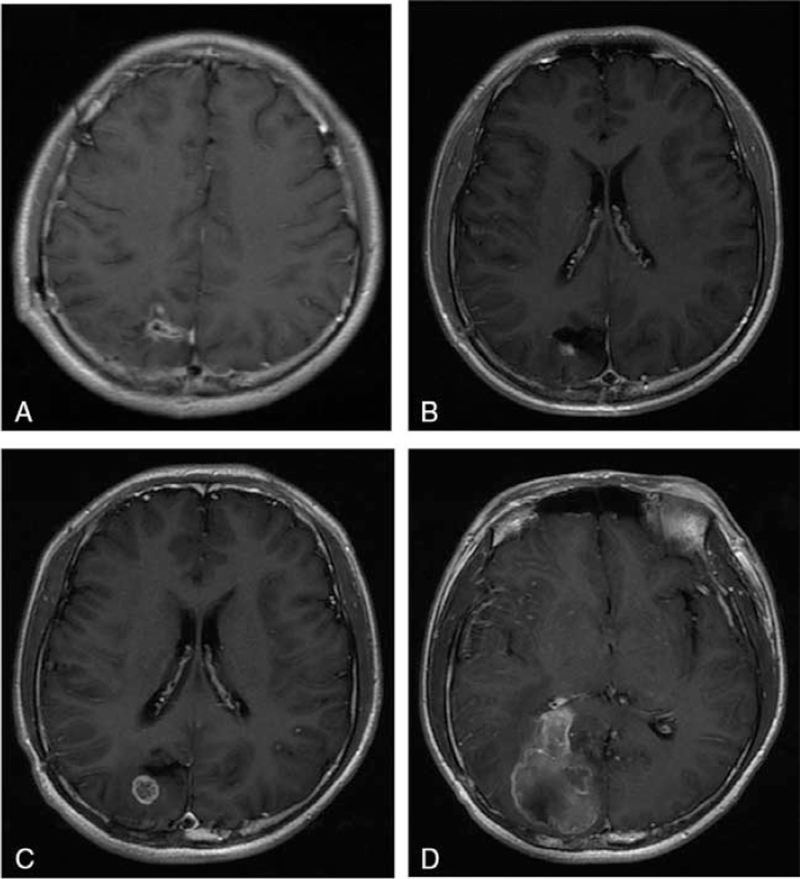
Postoperative imaging. A, Brain MRI obtained at first time after operative demonstrating gross total resection and consistent with favorable radiotherapy treatment response. B, Brain MRI showed nodular enhancement could be seen on the enhanced imaging. C, MR scan revealed increased lesion range, with evidence of tumor recurrence. D, Brain MRI obtained demonstrating enlargement of the tumor, with unclear boundaries and significantly heterogeneous enhanced. MRI = magnetic resonance imaging.
